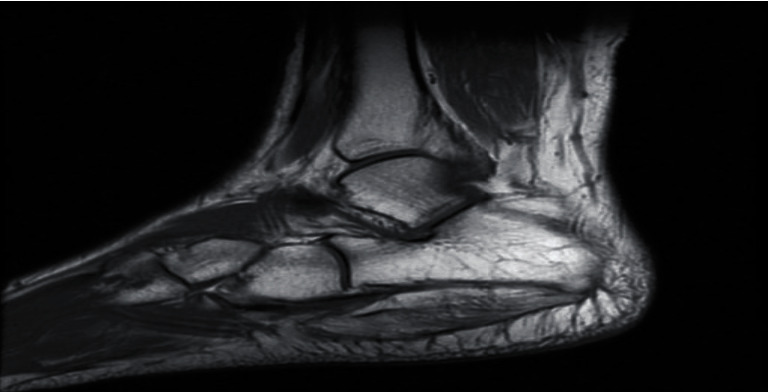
Magnetic resonance image of the right foot, Sag T1 showing diffuse enlargement of the muscle, with increased signal intensity. The muscle fibers are intact.
